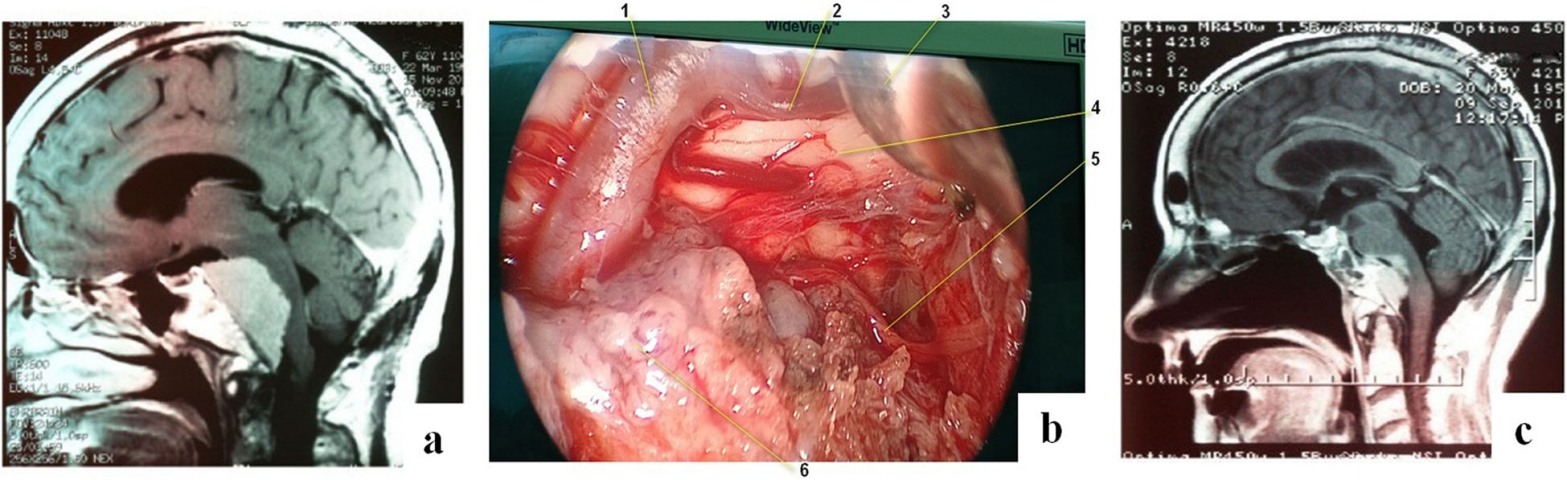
Case 3. Sixty-three-year-old female patient with a giant meningioma of the clivus and the left cerebellopontine angle. a MRI images before surgery. b Intraoperative photograph: 1—basilar artery; 2—left SCA; 3—aspirator; 4—upper pons; 5—left abducent nerve; and 6—tumor mass. c MRI images 4 months after surgery, subtotal removal of the tumor
